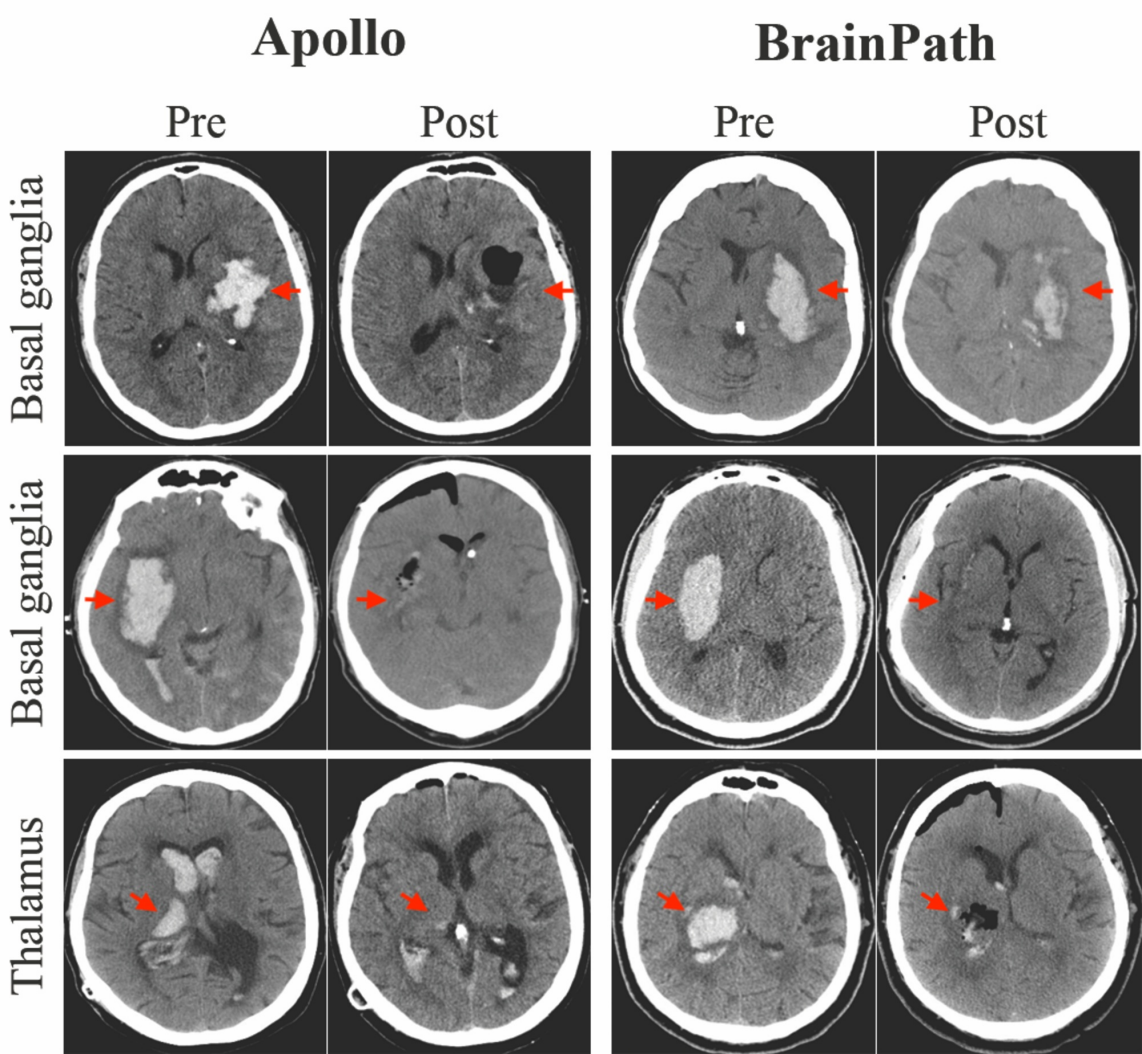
Pre- and postoperative axial CT images of the head of matched left basal ganglia, right basal ganglia, and right thalamic intracranial hemorrhages treated with the Apollo and BrainPath systems CT: Computed Tomography Apollo (Penumbra Inc, Alameda, California, US); Nico BrainPath (Indianapolis, Indiana, US)
